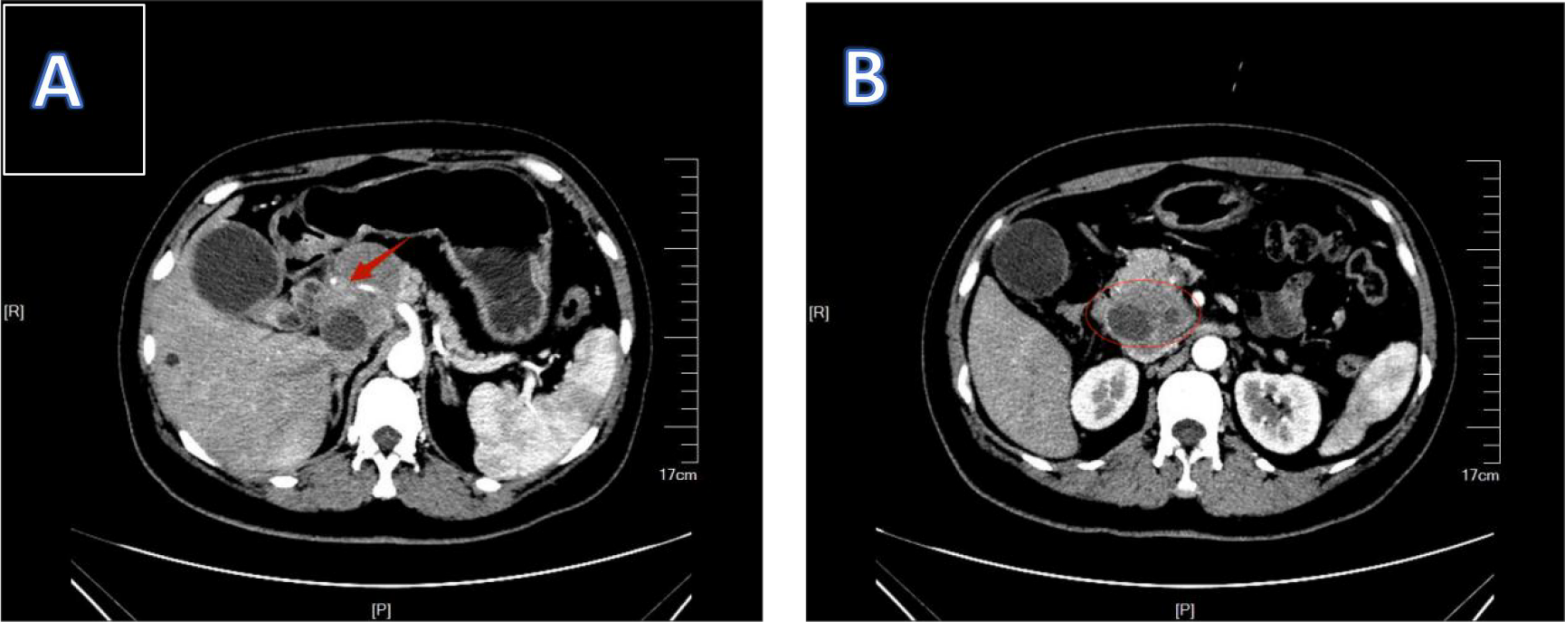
2Patient 1’s enhanced CT results. (A) shows that the lymph nodes are surrounding the hepatic artery. (B) shows enlarged lymph nodes.
